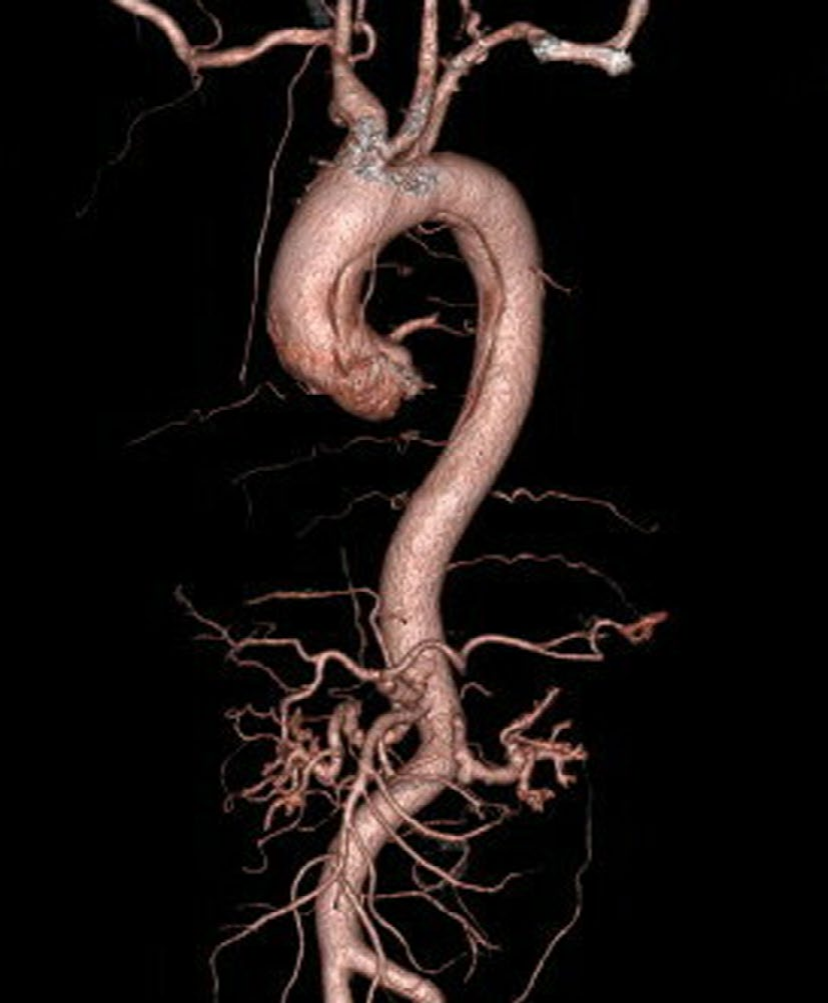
Preoperative 3D computed tomography imaging showed aortic dissection from ascending aorta to descending aorta
